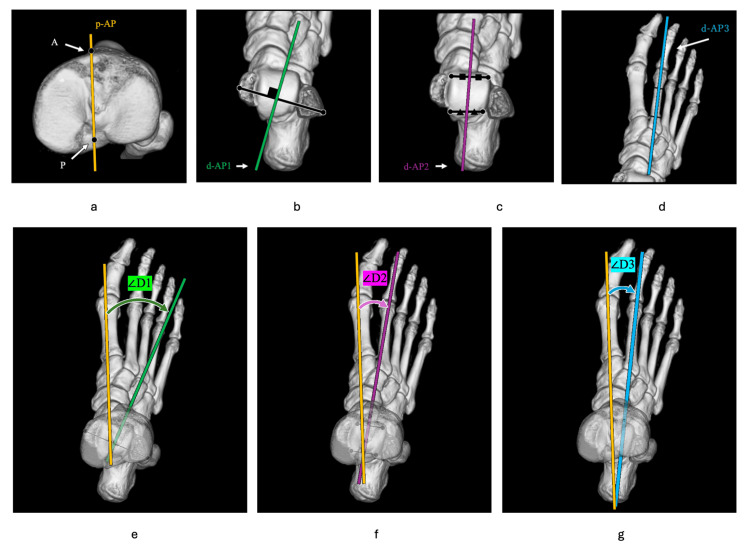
Definition of axes and angles on 3D reconstructed CT a) The p-AP axis was defined as the line connecting the medial border of the tibial tuberosity (A) and the center of the posterior cruciate ligament attachment (P). b) The d-AP1 was defined as the AP axis of the ankle joint. c) The d-AP2 was defined as the AP axis of the talus. d) The d-AP3 was defined as the second metatarsal bone axis. e, f, g) The angles of the d-AP1 (e), d-AP2 (f), and d-AP3 (g) to the p-AP were defined as ∠D1, ∠D2, and ∠D3, respectively. All measurements were performed with an external rotation direction of +.
